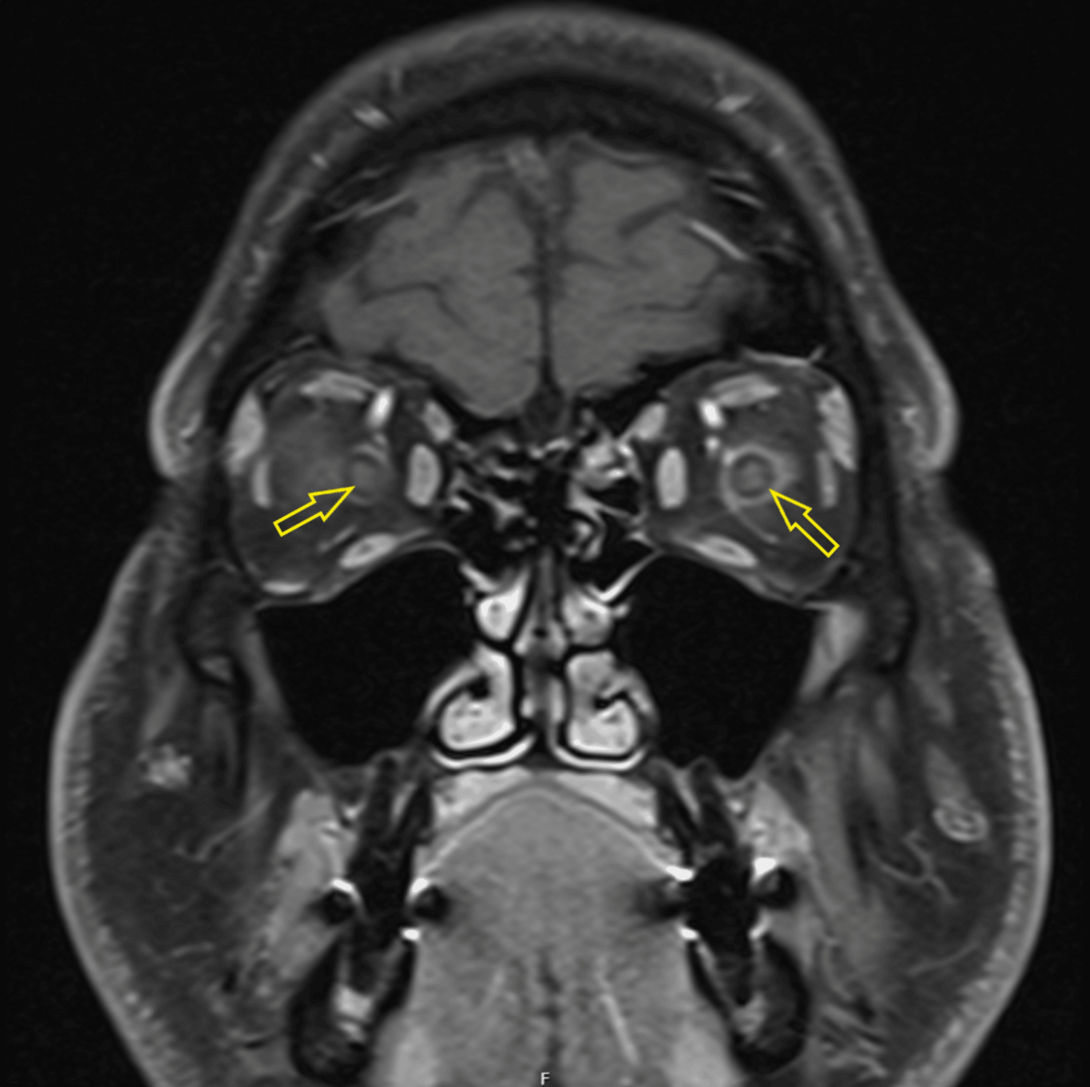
Post-contrast T1-weighted coronal MRI image showing left greater than right optic nerve sheath contrast enhancements and thickening, consistent with perineuritis.
